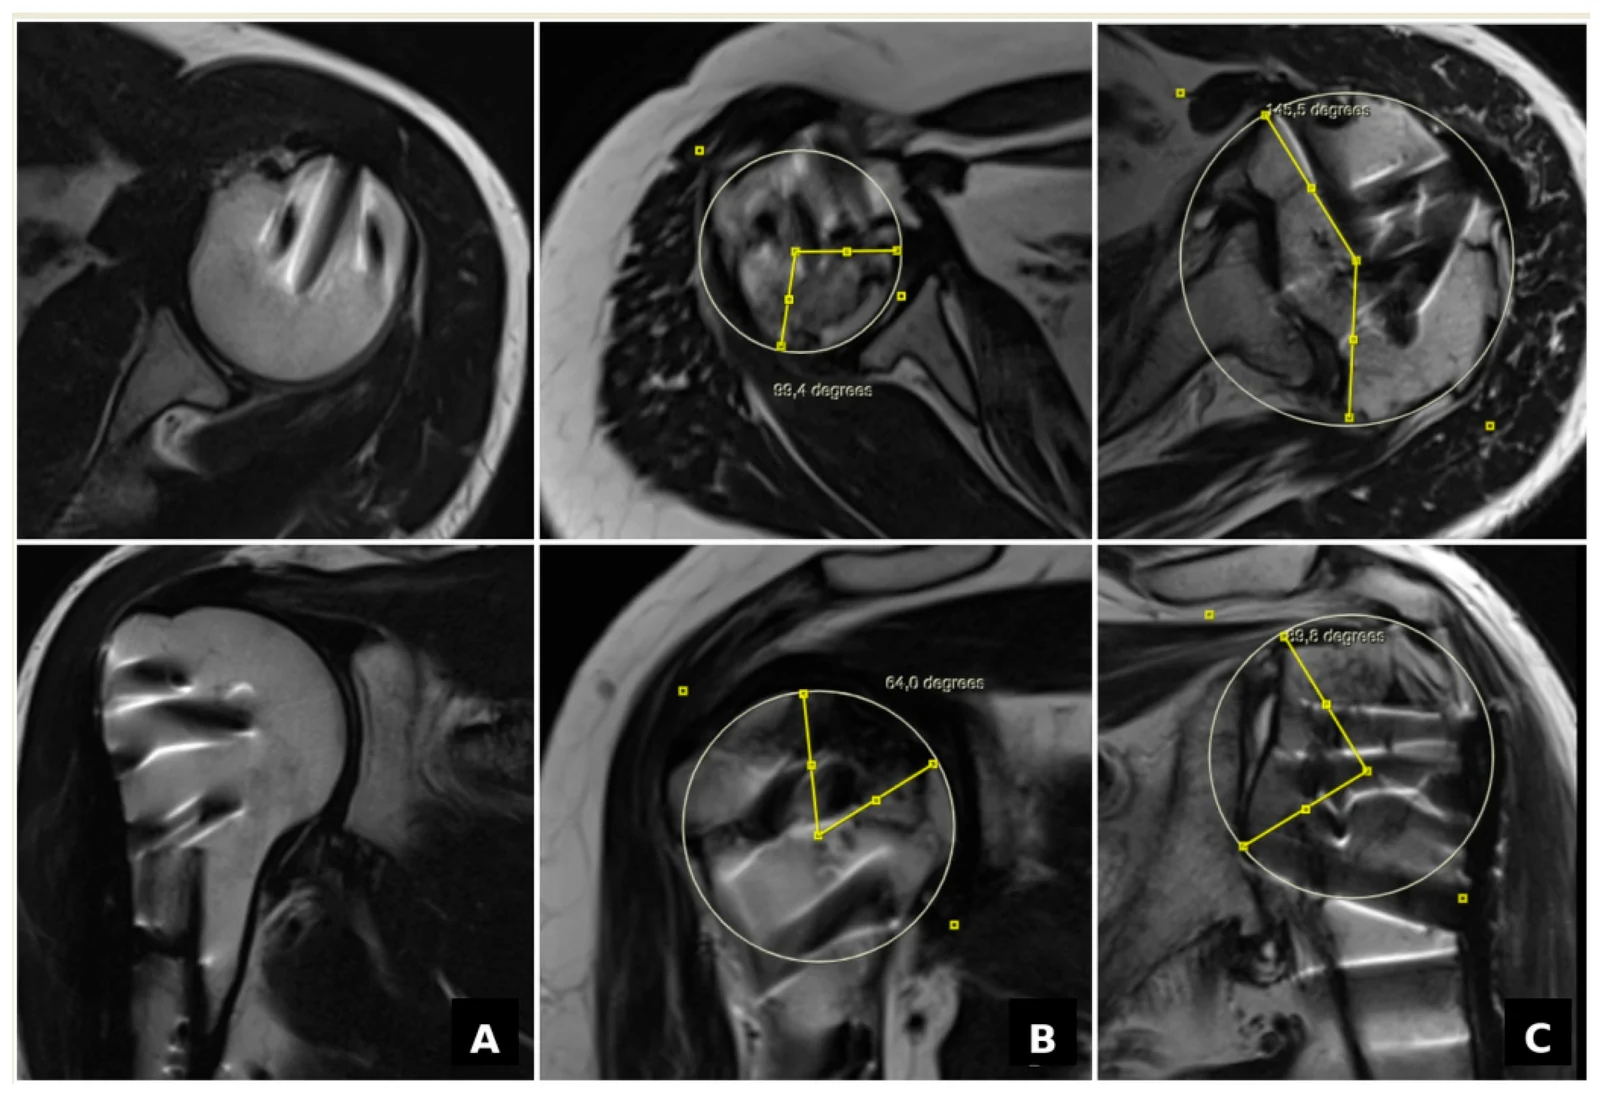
MRI-based quantification of humeral head osteonecrosis extent after locked plate fixation of proximal humeral fractures. (A) Fifty-eight-year-old man, Cruess stage I osteonecrosis. Mid-coronal T1-weighted image demonstrating preserved humeral head morphology without measurable necrotic angle. (B) Forty-seven-year-old woman, Cruess stage III osteonecrosis, total necrotic angle 163° (coronal 99°, sagittal 64°). Mid-axial fat-suppressed proton density-weighted image showing the necrotic angle measurement. (C) Sixty-three-year-old man, Cruess stage IV osteonecrosis, total necrotic angle 235° (coronal 146°, sagittal 90°). Representative coronal and sagittal images demonstrating a large necrotic lesion with advanced structural involvement. Total necrotic angle was calculated as the sum of the coronal and sagittal plane measurements. Yellow lines and circles indicate the best-fit circle and angle measurement lines used to quantify the necrotic angle on each imaging plane.
